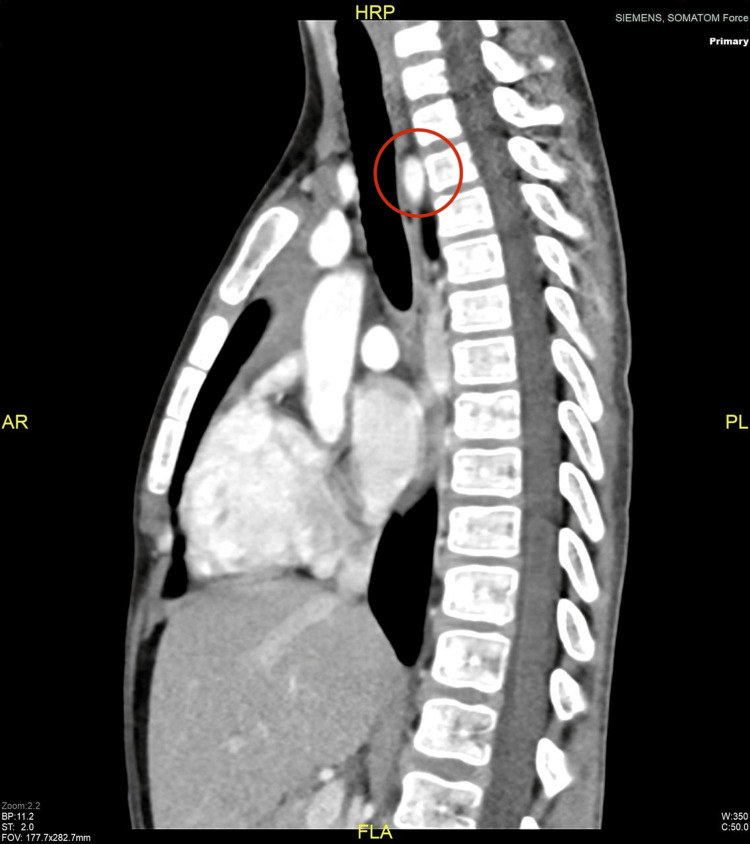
Sagittal view of CT angiography revealing an aberrant right subclavian artery (red circle) coursing retroesophageally.
